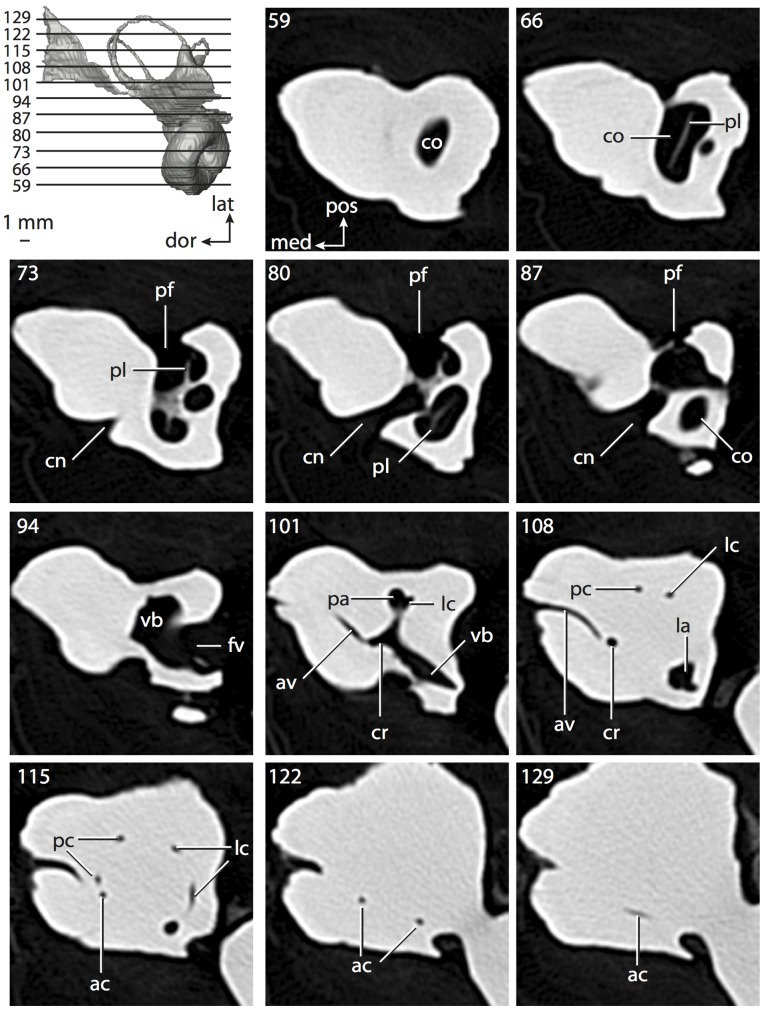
CT slices through ear region of*Trichechus manatus*. Abbreviations listed at the end of the Materials and Methods section.
